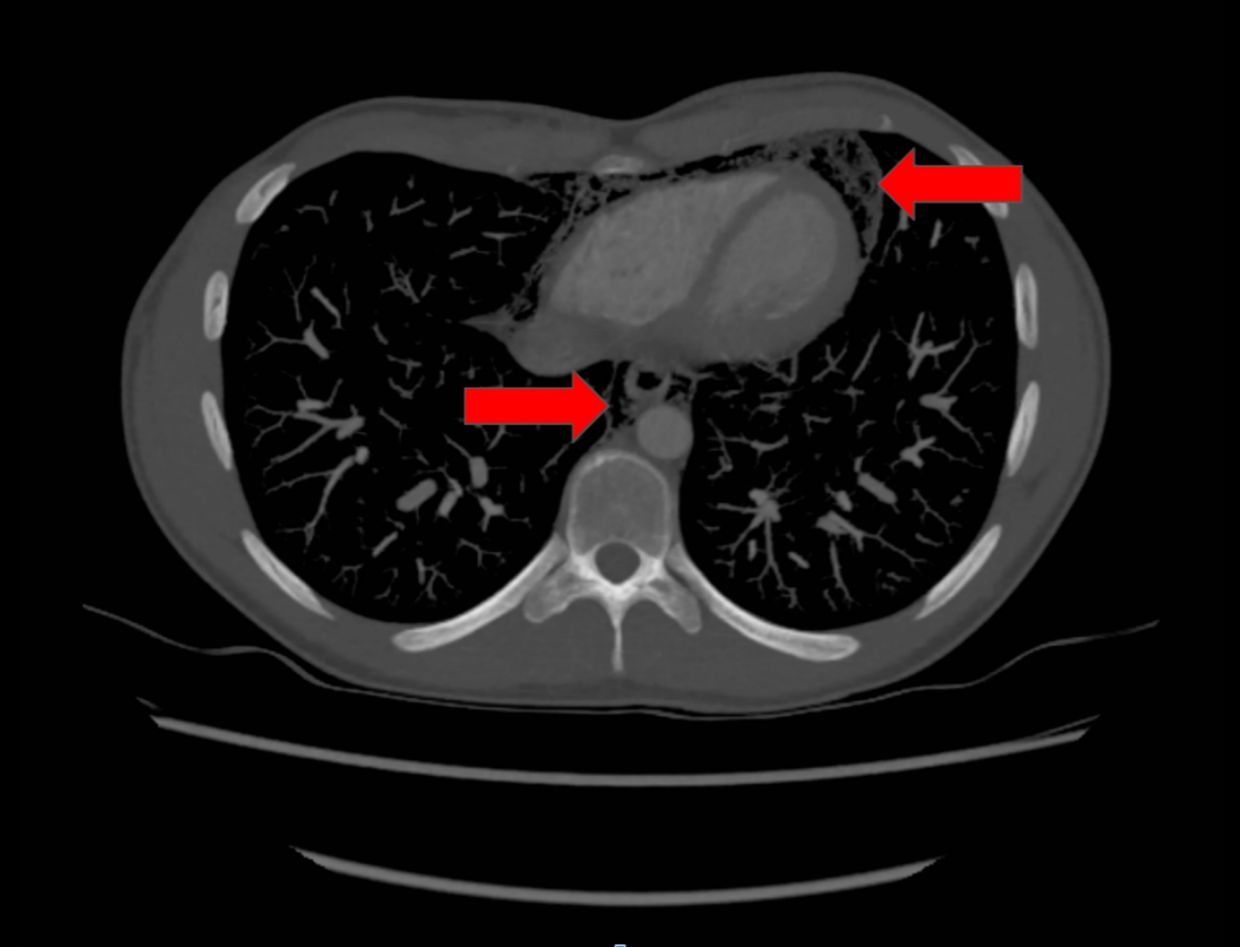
Transverse computed tomography (CT) showing lower air in the lower mediastinum and around the pericardium (red arrows)
